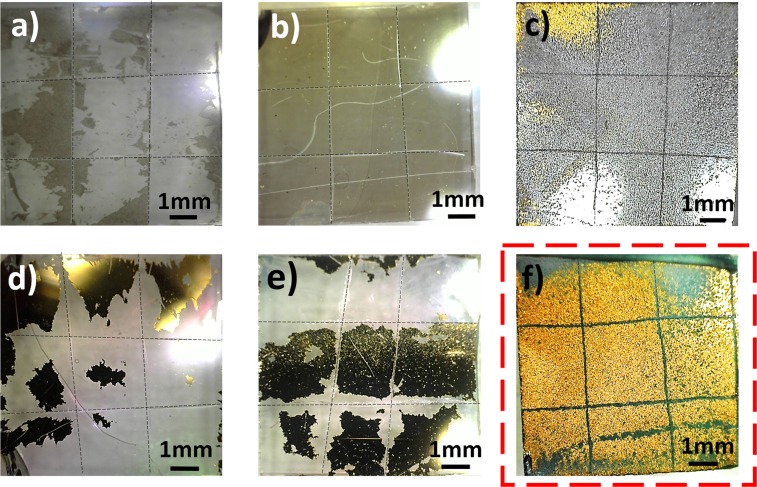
Sample surface image after applying ultrasonic test. (a) TE (b) HF-TE (c) Rough-TE (d) Sputt (e) HF-Sputt, and (f) Rough-Sputt.
